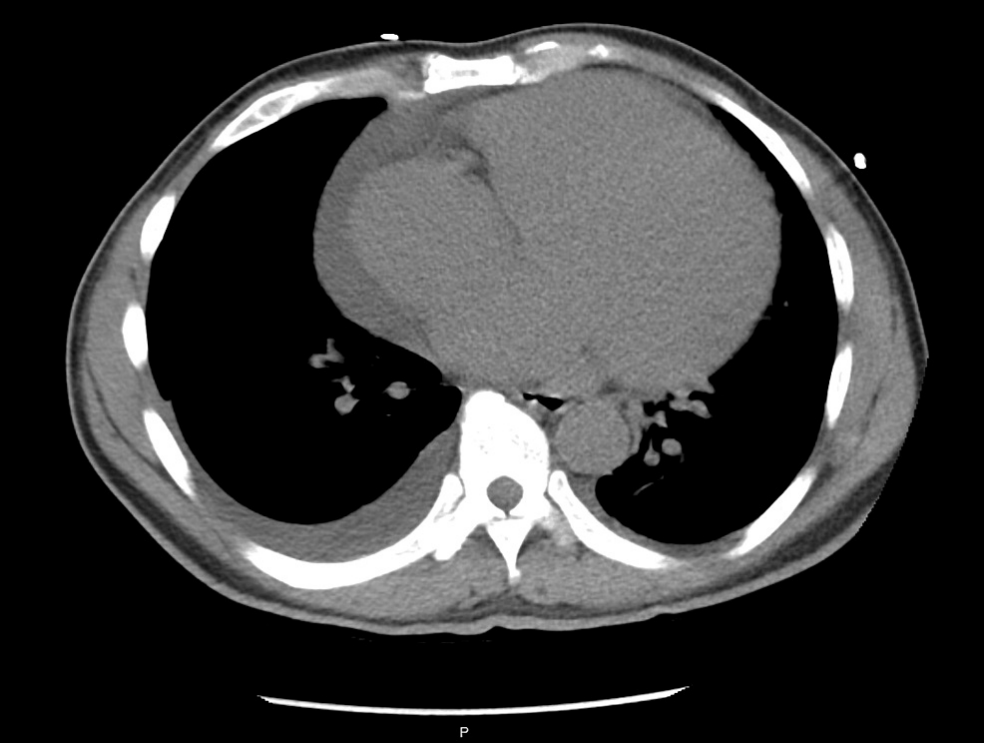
CT angiogram of the chest reveals severe cardiomegaly, a moderate pericardial  effusion, and bilateral pleural effusions.
